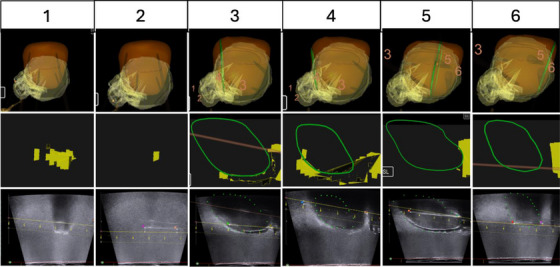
Top) 3D‐rendered uterus (orange) and CTV_HR_ (yellow) with needle target locations indicated; Middle) the contour of CTV_HR_ (solid yellow) and uterus (green) in the plane of the TRUS transducer when rotated to the target point of interest; Bottom) live TRUS image with the intended needle path (solid yellow) displayed in the same plane as the actual needle insertion. TRUS, transrectal ultrasound.
